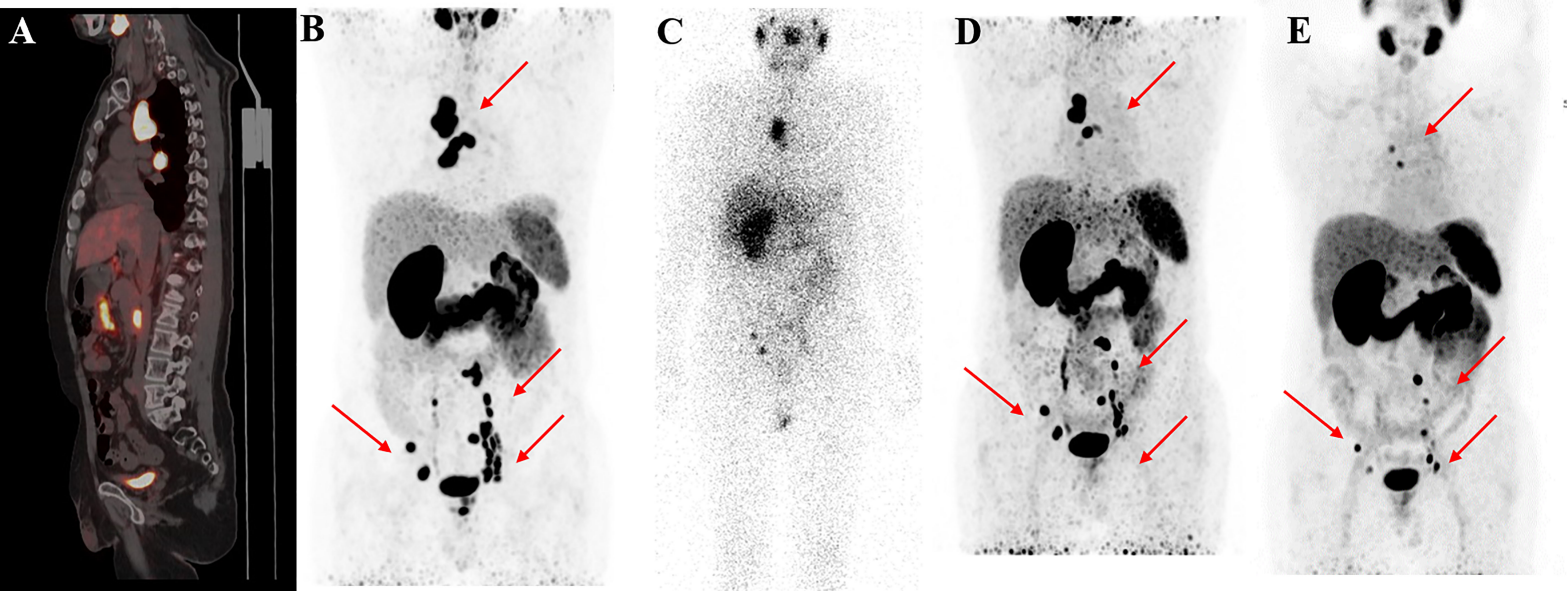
^68^Ga-PSMA-11 PET/CT in a 55-year-old patient who underwent four cycles of ^177^Lu-PSMA-I&T therapy. Baseline images (A, B) of multiple lymph node metastases in the right subclavian area, mediastinum, retroperitoneum, and pelvis. Post-therapy image (C) showing higher ^177^Lu-PSMA-I&T aggregation in primary and metastatic lesions. ^68^Ga-PSMA PET/CT after the first cycle (D) and after the third cycle (E) showing multiple decreased or disappeared metastases.
